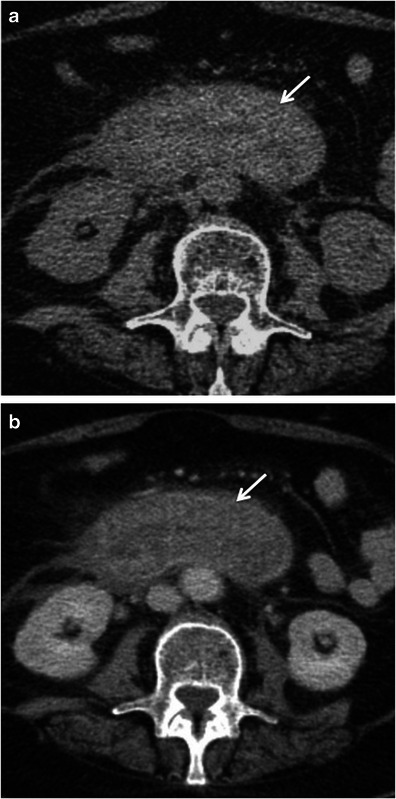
White attenuation pattern due to spontaneous intramural bowel wall haemorrhage in a patient with polyarteritis nodosa. Axial unenhanced (a) and contrast-enhanced (b) CT scans of the abdomen show concentric wall thickening of the third duodenal portion that showed spontaneous hyperenhancement of the bowel wall due to spontaneous haemorrhage. *Adapted from the electronic poster “Bowel wall thickening—a complex subject made simple” DOI:*10.5444/esgar2011/EE-063
